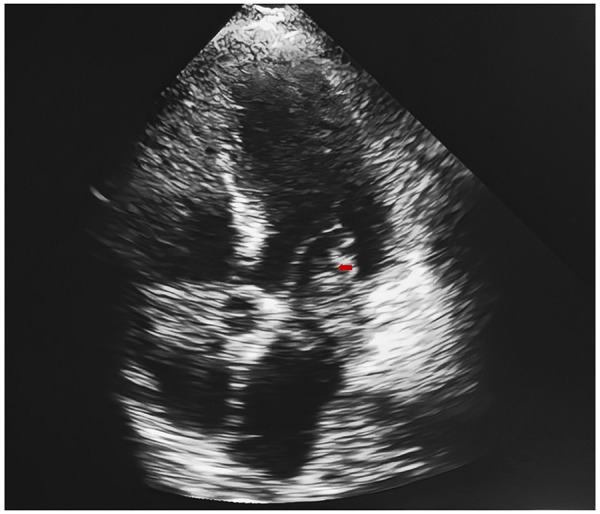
Transthoracic echocardiography shows an echogenic lesion in the left atrium, as indicated by the red arrow.
